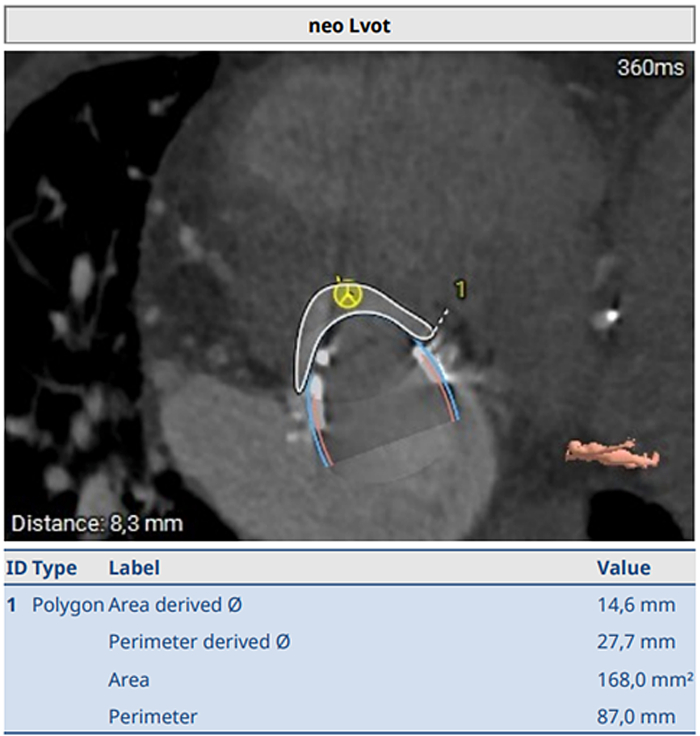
Computed Tomography Assessment of Neo-LVOT Before Mavacamten Computed tomography–based measurement of the predicted neo-LVOT area before mavacamten therapy, measuring 168 mm^2^. LVOT = left ventricular outflow tract.
